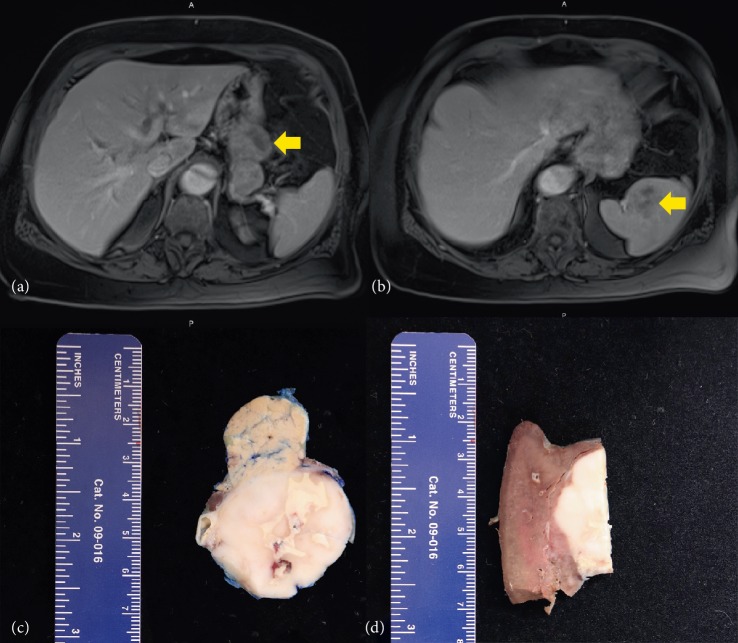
(a and b) showing contrast-enhanced T1 MR images of the pancreatic (a) and splenic (b) lesions, arrows point to the lesions; (c) shows the gross image of the IPT-like FDCT in the pancreas; (d) shows the gross image of the IPT-like FDCT in the spleen.
